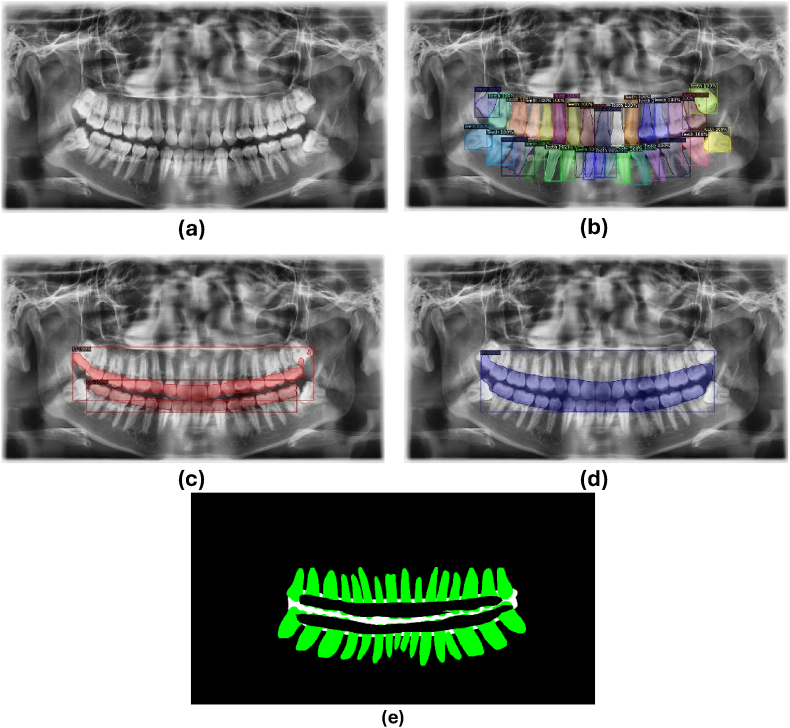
(a) A sample of panoramic radiograph from the dataset, with the results of segmentation training indicating the detection of (b) teeth, (c) the cementoenamel junction (CEJ), and (d) radiographic bone loss (RBL) on the same image. (e) Mask from the segmentation training, which serves as input for the staging training.
